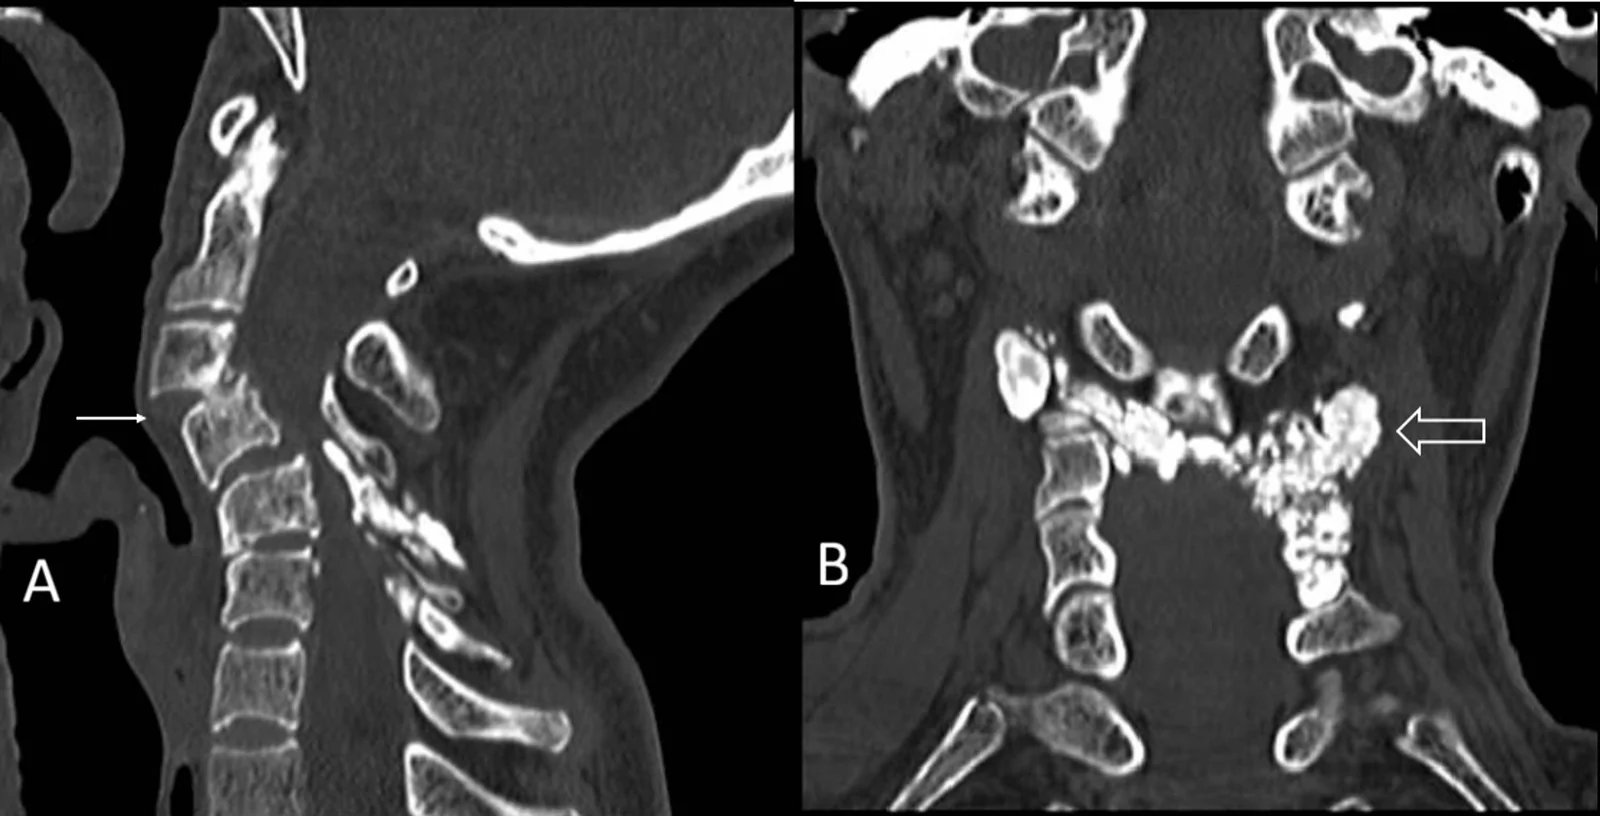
Computed tomography scan: (A) mid-sagittal and (B) coronal views demonstrating multilobulated calcified masses centered on the C3-C6 facet joints and posterior elements (hollow arrow), with associated facet destruction and anterolisthesis at C3-4 and C4-5 (solid arrow).
